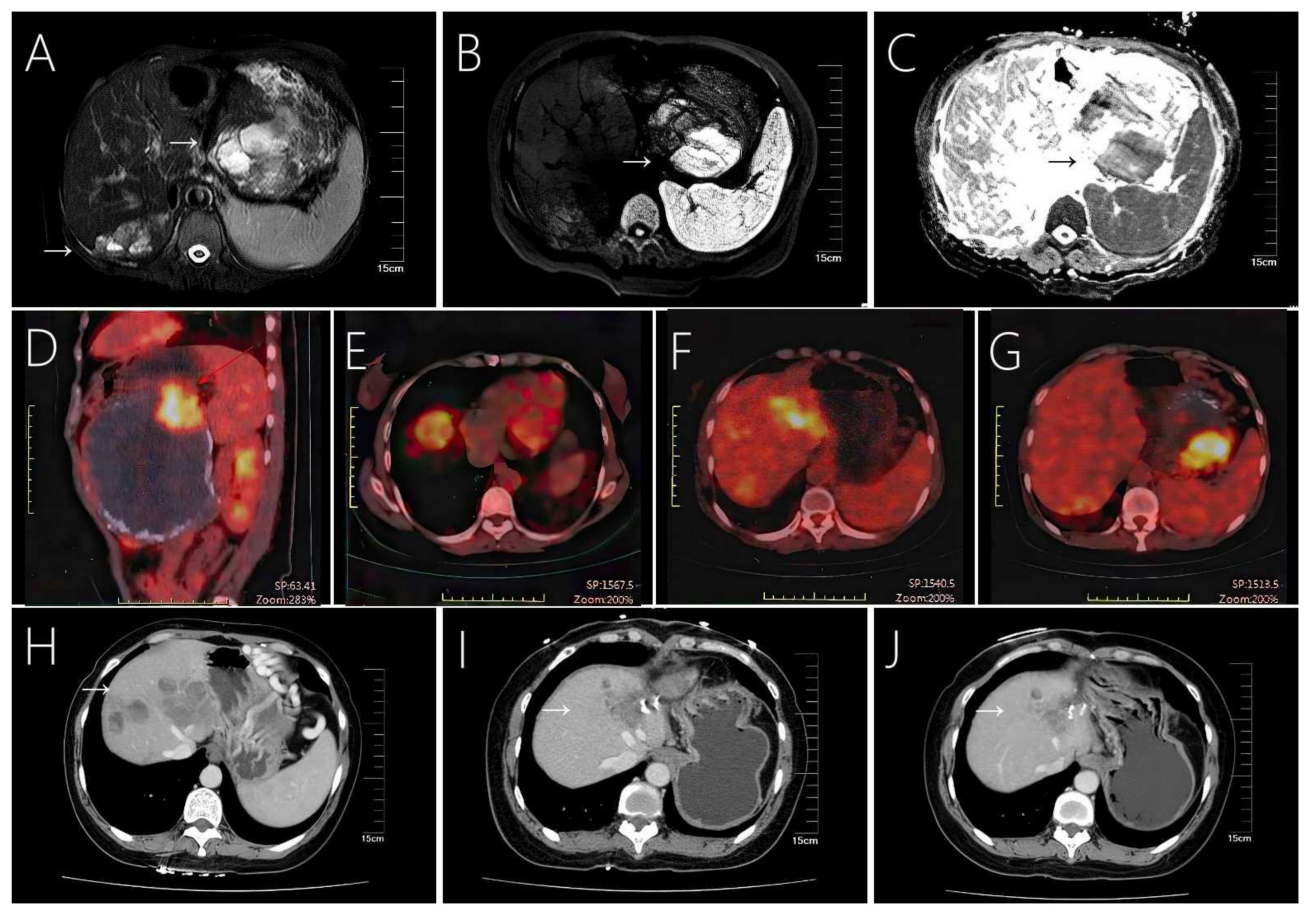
Case 8 shows a large solid pseudopapillary tumor in the tail of the pancreas with extensive multiple liver metastases, (A) showing a mixed cystic and solid structure. (B, C) MRI shows marked diffusion restriction within the primary tumor. (D–G) Tumors exhibit eggshell-like calcifications and increased radioactive uptake on PET-CT. Enhanced CT scan showed that the patient’s liver metastases existed in the same part of the tumor in the case of resection of the primary tumor in the patient (H) before surgery, (I) 20 months after surgery, and (J) 28 months after surgery, decrease or disappear.
